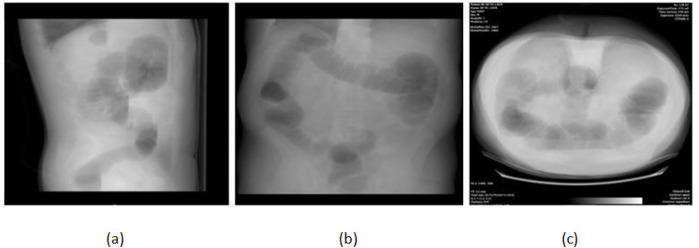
The 2D X-Ray Image Created from a Dataset of 1000 CT Images of Abdomen Scan which Shows the Large Intestine Distribution (C=-200, W=1500), A. Left-Right view, B. Anterior-Posterior view, and C. Inferior to Superior view
